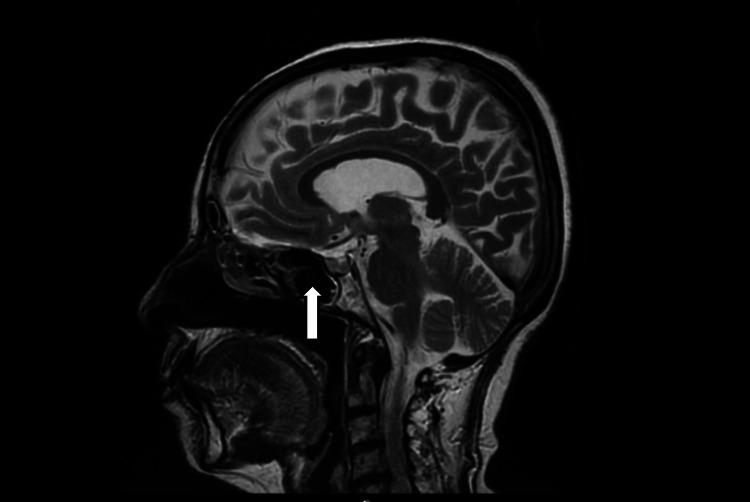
T2-weighted MRI in the mid-sagittal section that demonstrates a fluid-filled sella turcica with a flattened pituitary that is just visible at the base of the pituitary fossa
